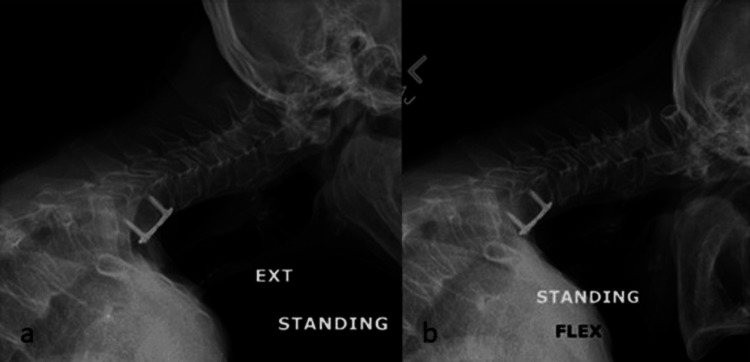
(a) X-ray of patient in extension four months post DHS onset; (b) X-ray of patient in flexion four months post DHS onset.
